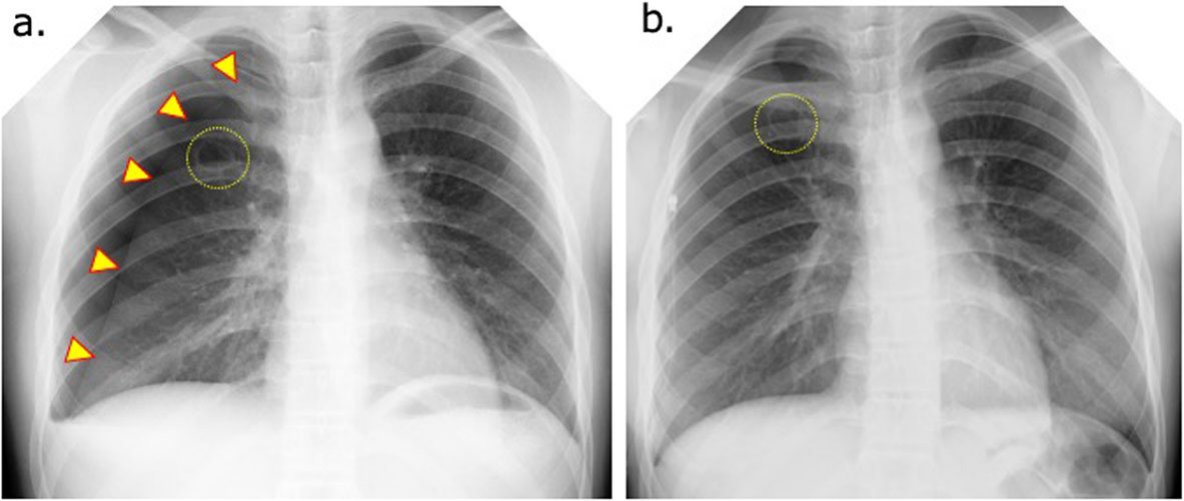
Chest X-ray at the patient’s first consultation. a Right pneumothorax is seen (yellow arrowheads). b At the previous hospital, placement of a thoracic drain for right pneumothorax did not improve the pneumothorax. Cyst wall thickening is visible in the upper right lung field (dashed yellow circle)
